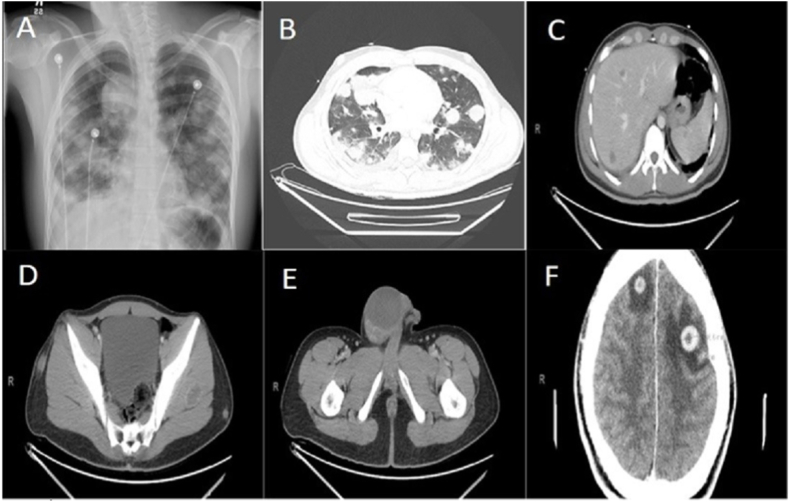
A: Bilateral diffuse rounded opacities with predominant with predominant right supra-hilar mass. B: CT chest with contrast showed bilateral lung nodule and masses with predominant mass in right upper lobe/mediastinum. C: CT abdomen showed hepatic and splenic metastases. D: CT Pelvis showed left gluteal mass, which was biopsied. E: CT pelvis showed homogenous right testicular mass. F: CT head showed bilateral hemorrhagic brain metastases.
